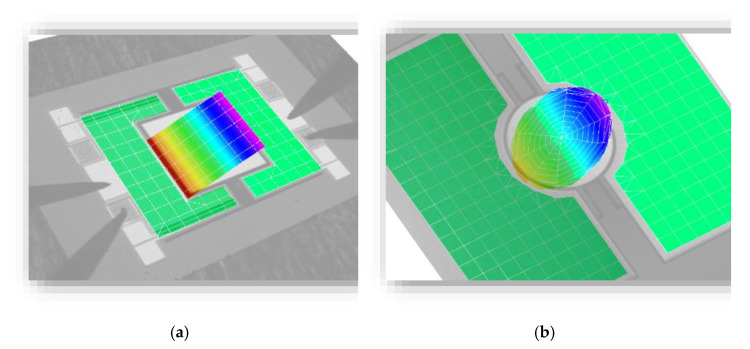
Motion scan images of exemplary micromirrors recorded by Laser-Doppler-Vibrometry: (a) Design 1; and (b) Design 2.
